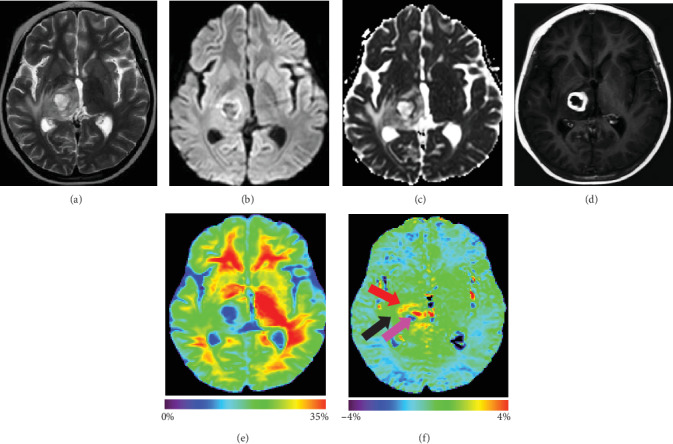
APTw, MTR, and conventional MR images for a 7-year-old girl with tuberculous abscess. T2w image (a) shows an irregular lesion with surrounding edema in the right thalamus. DWI (b) and ADC map (c) show restricted diffusion in part of the lesion. Gd-T1w image (d) demonstrates an enhancing rim with a nonenhancing necrotic center. The MTR (e) signals are low in the gadolinium-enhancing rim of the lesion and perifocal edema. APTw image (f) shows that the enhancing rim (red arrow) is hyperintense, while the necrotic region (pink arrow) and perifocal edematous area (black arrow) have low and equal APTw signals.
